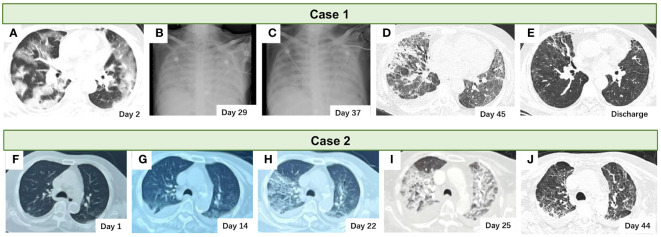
The pulmonary radiological images of the two cases. (A–E) The chest CT scan and the X-ray of the case 1 patient. (F–J) The chest CT scan of the case 2 patient.
